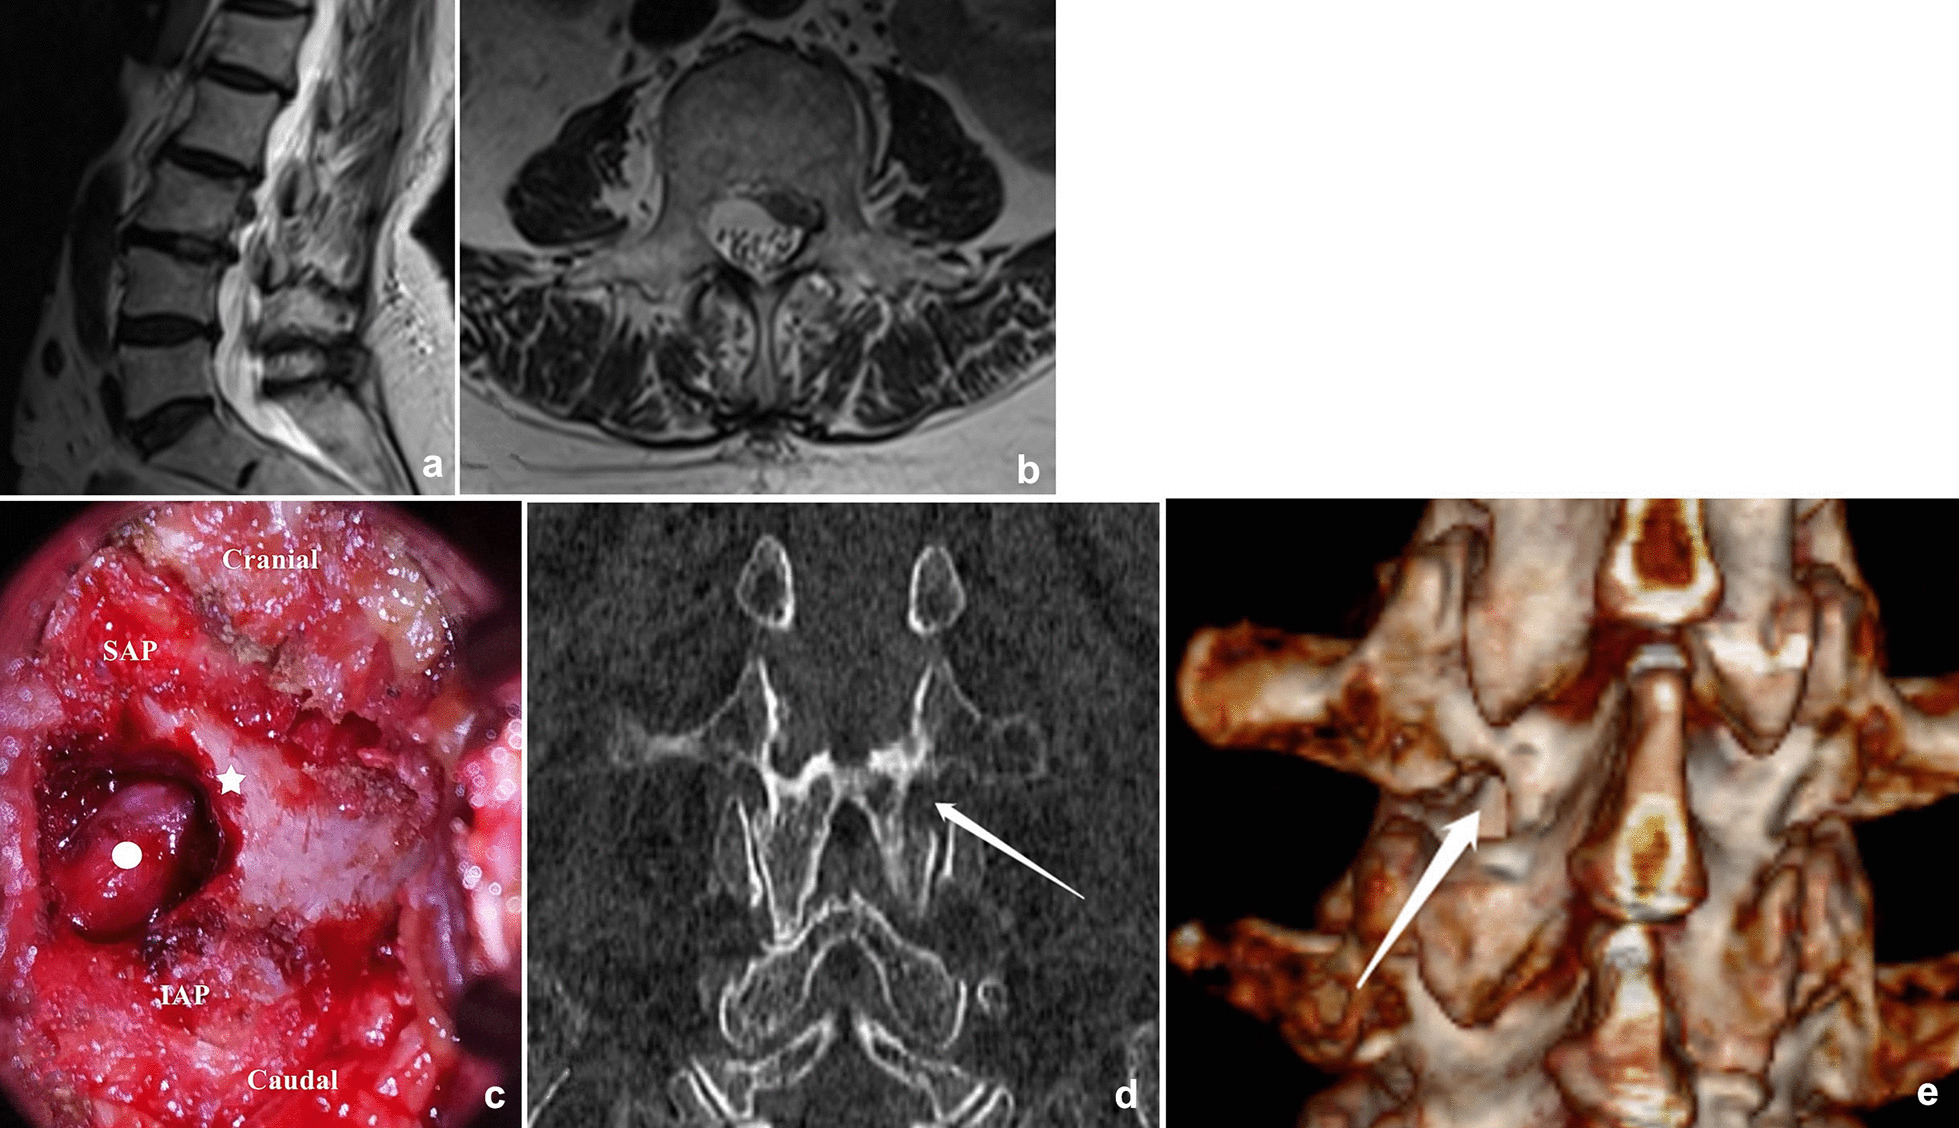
a, b The preoperative MRI showed a complete sequestered fragment. c The intra-operative view: the exiting nerve root was exposed after the “cresent-shaped” excision of the lamina (white circle: exiting nerve root; white star: lateral margin of the lamina; SAP: superior articular process; IAP: inferior articular process). d, e immediate postoperative CT shows the crescent-shaped excision of the lateral lamina (white arrow)
